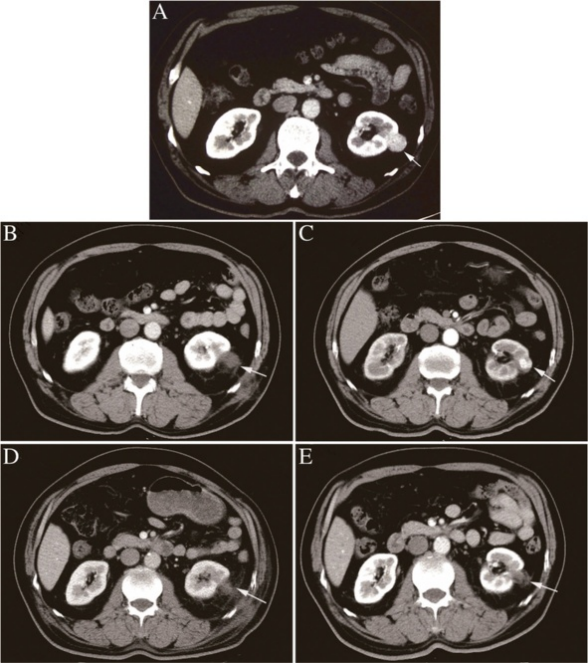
Sequential contrast-enhanced CT images of the ablation area of renal cell carcinoma (RCC) measuring 2.8 cm observed for the longest period with two cryoablations. a Contrast-enhanced CT before cryotherapy. b Contrast-enhanced CT 1 month after stage 1 cryotherapy. c Contrast-enhanced CT 12 months after stage 1 cryotherapy. d Contrast-enhanced CT 1 month after stage 2 cryotherapy. e Contrast-enhanced CT 12 months after stage 2 cryotherapy
